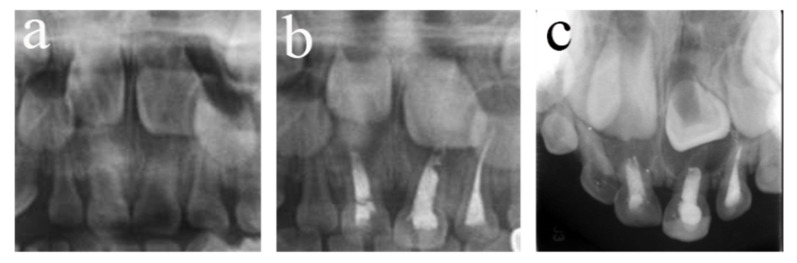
#51, #61, and #62 received pulpectomies under DGA with a 12-month follow-up period (57-month-old female child). (a) Preoperative radiograph and #61 revealed periapical radiolucency. (b) Two weeks later, the postoperative radiograph showed underfilling in #61 and optimal filling in #51 and #62. (c) Radiograph taken 12 months postoperatively showing nothing abnormal about #62 and a periapical radiolucency surrounding the root apical of #51. Moreover, a radicular cyst occurred surrounding the apical root of #61, and the eruption direction of #21 was altered. Pulpectomies in #62 succeeded but failed in #51 and #61.
